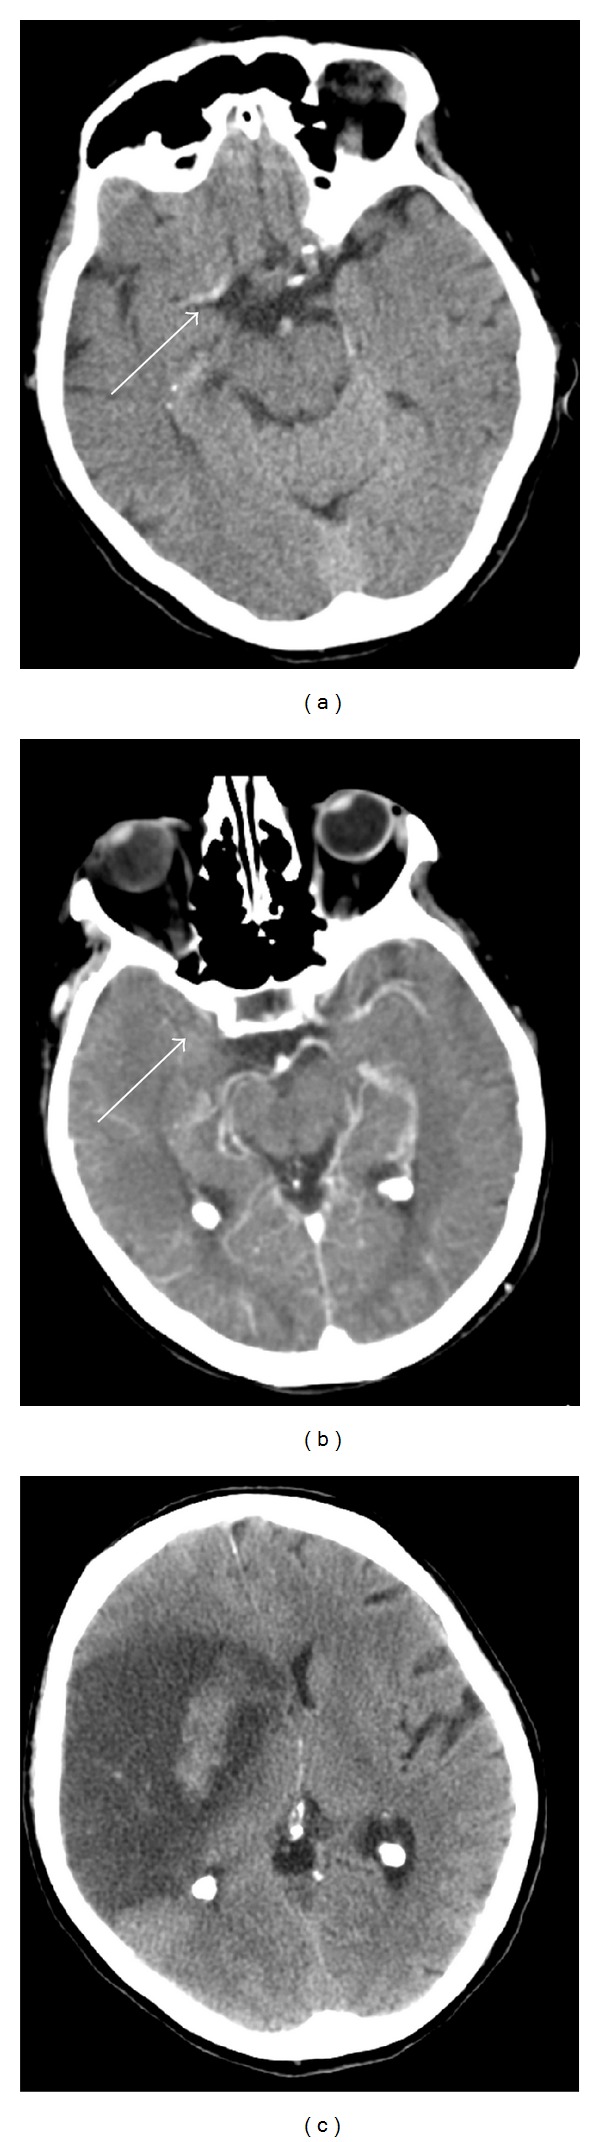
Imaging of initial ischemic stroke. (a) CT without contrast, showing dense right MCA (white solid arrow) and loss of differentiation of the grey-white junction on the right. (b) CT angiography, revealing occlusion of the right MCA (white solid arrow). (c) CT without contrast, showing subsequent hemorrhagic transformation of the infarct.
